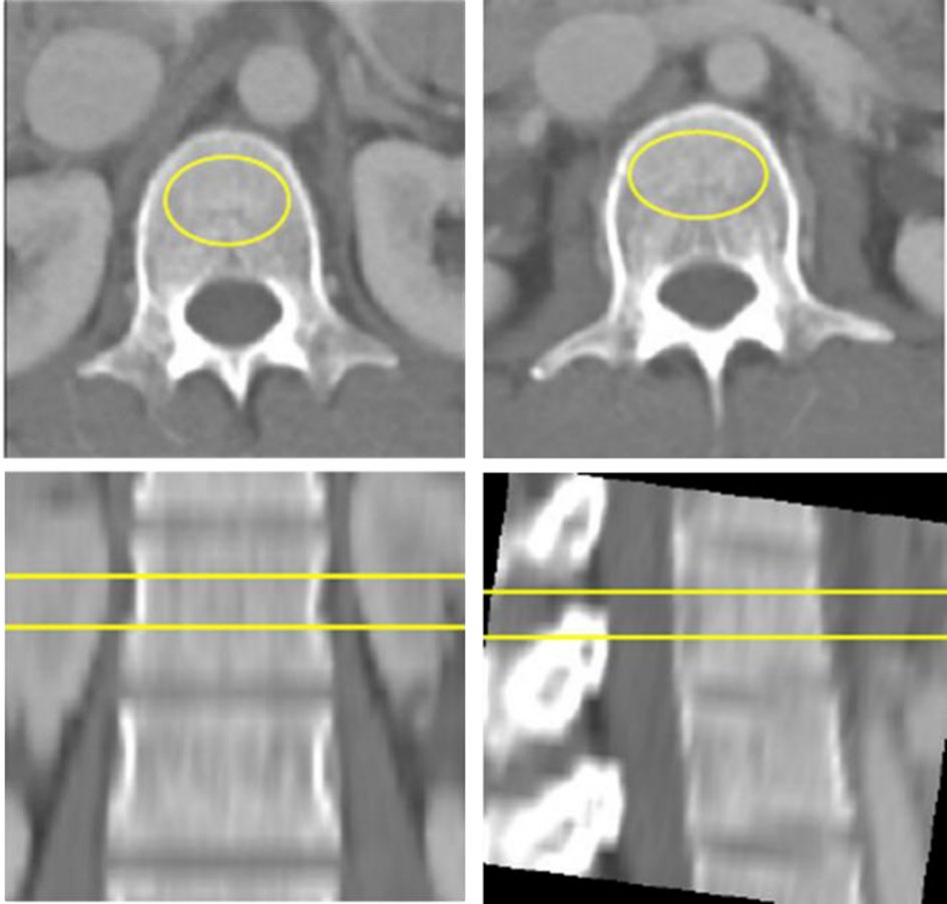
Manually measuring v-BMD on abdominal CT using asynchronous QCT. v-BMD = volumetric bone mineral density, QCT = quantitative computed tomography.
